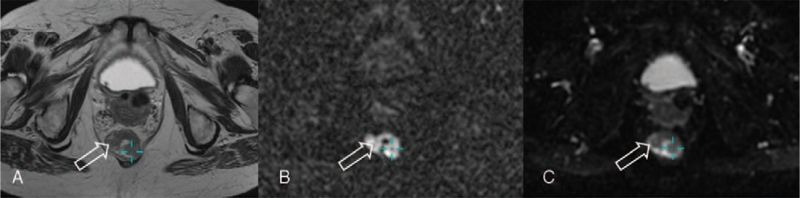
Axial T2-weighted (A), diffusion-weighted (DWI) b = 1000 s/mm^2^ (B) and apparent diffusion coefficient (ADC) map (C) magnetic resonance images. Diffusion-weighted magnetic resonance images suggested the malignant character of the thickened (A) rectal and anal canal wall. High signal on B-value diffusion-weighted images (B) with corresponding low signal on ADC maps (C) confirmed water diffusion restriction – a sign of malignancy (hollow arrows).
